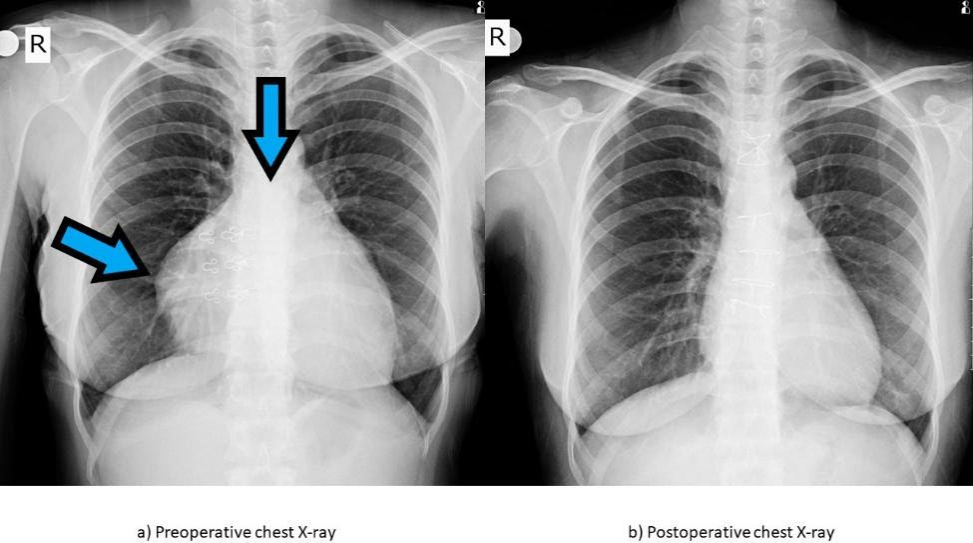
(a) Preoperative chest X-ray showing the mass in the right pericardiac and mediastinal area. (b) Postoperative chest X-ray showing the shrinking mass
